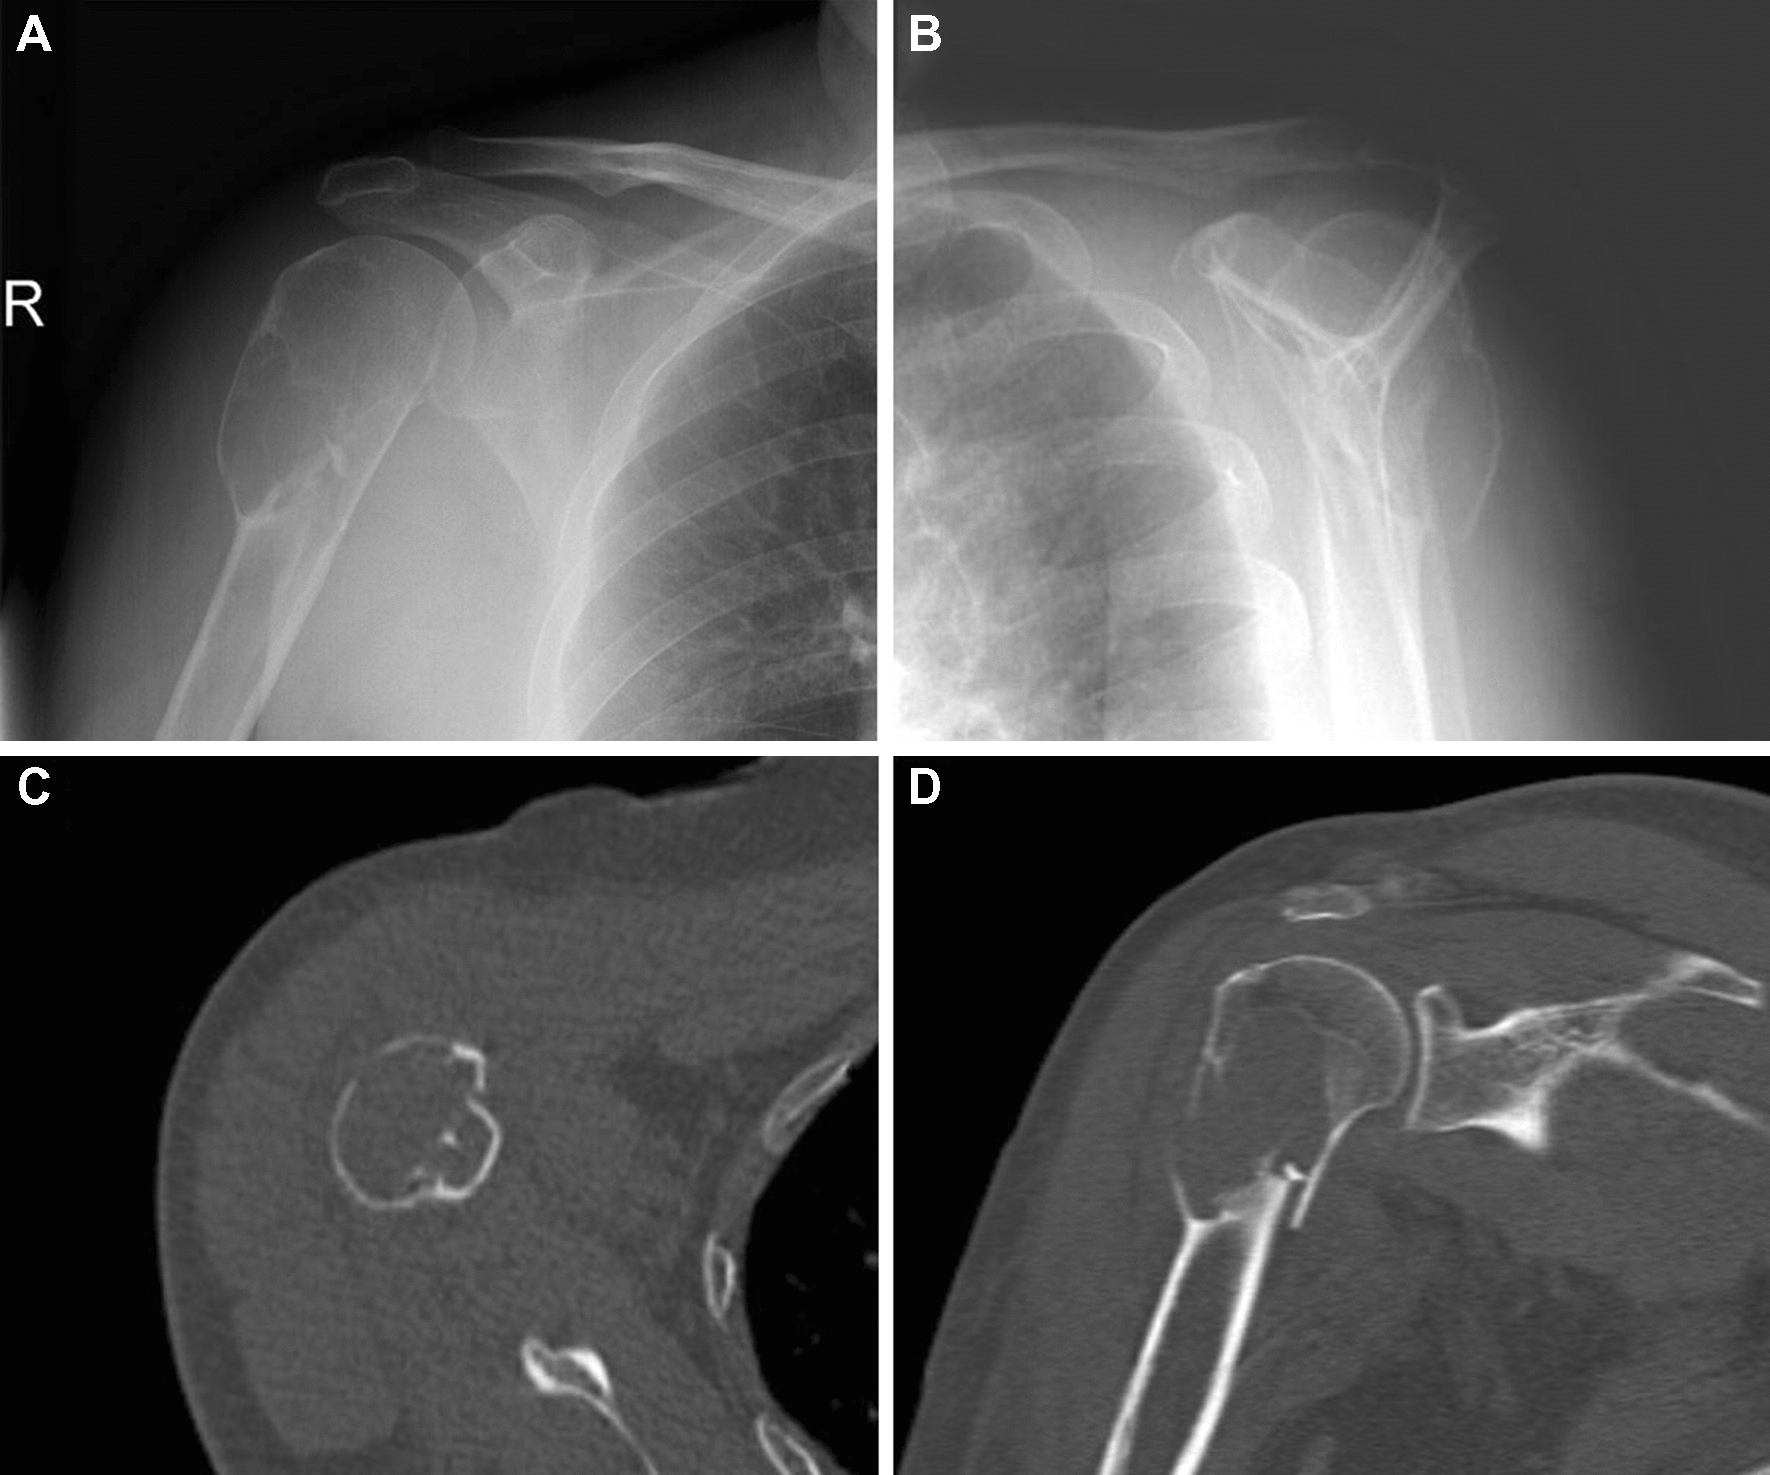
Anteroposterior (A) and lateral (B) radiographs, shows an expanding, lytic lesion affecting the proximal humerus associated with scalloping of the endosteum. Computed tomography (CT) axial slice bone window (C) shows a intramedullary mass elevating the periosteum. Coronal slice bone window (D) shows a fracture at the proximal humerus region
